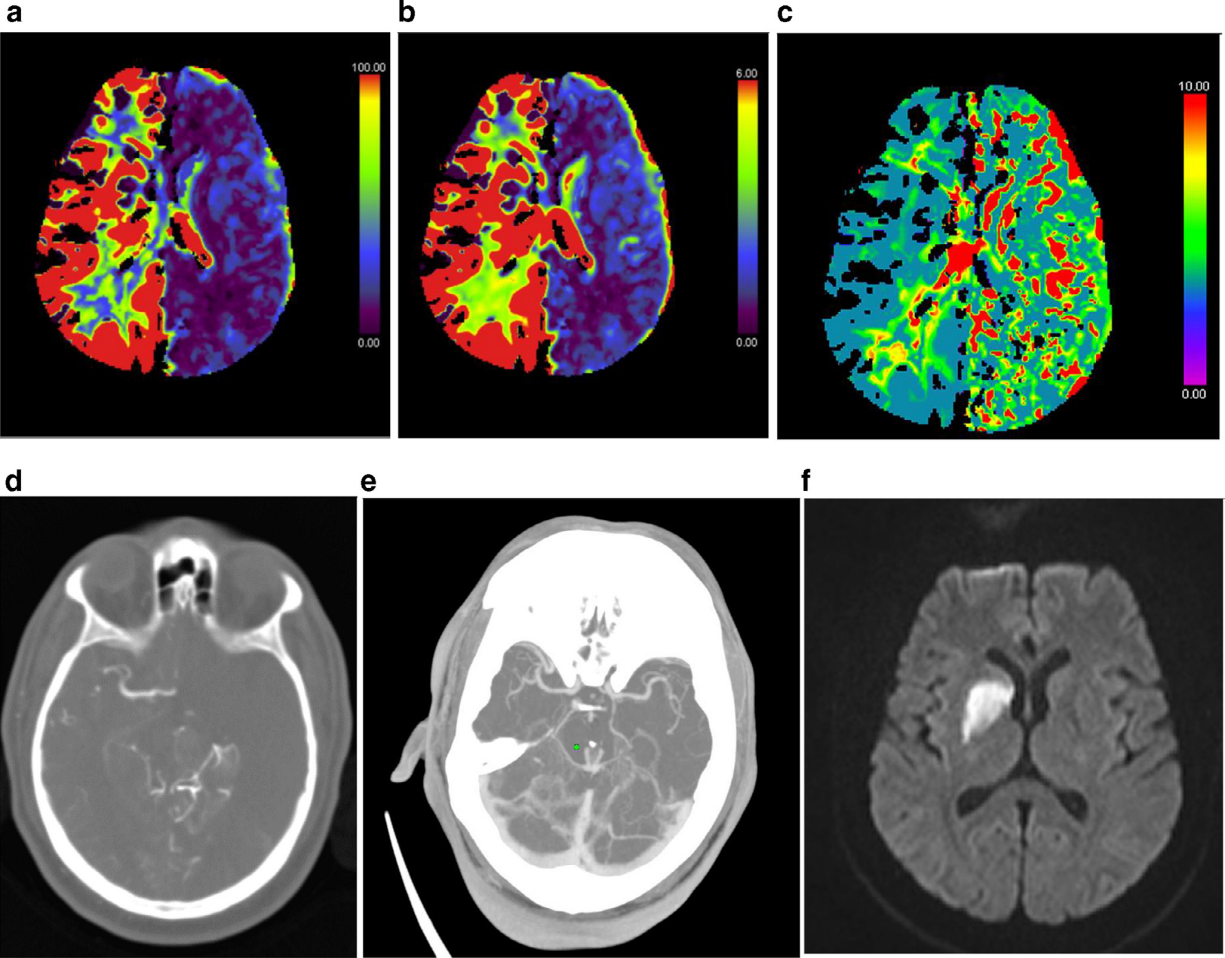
(A): Cerebral Blood Flow (CBF) in CT perfusion showing no flow in the in the left cerebral hemisphere with normal flow in the right cerebral hemisphere. (B): Cerebral Blood volume (CBV) in CT perfusion showing no volume the in the left cerebral hemisphere with normal volume in the right cerebral hemisphere. (C): CT perfusion (Mean Transit Time MTT) shows increased transit time in the left cerebral hemisphere. (D): CT perfusion Raw images (volume perfusion computed tomography (VPCT) DynMulti4D)- showing flow seen in the right middle cerebral arteries with absent left middle cerebral artery circulation. (E): CT angiography maximum intensity projection (MIP) images: Show normal flow in both middle cerebral artery territories. (F): MRI, Diffusion weighted images show diffusion restriction in the right basal ganglia region with no corresponding diffusion restriction in the left cerebral hemisphere.
